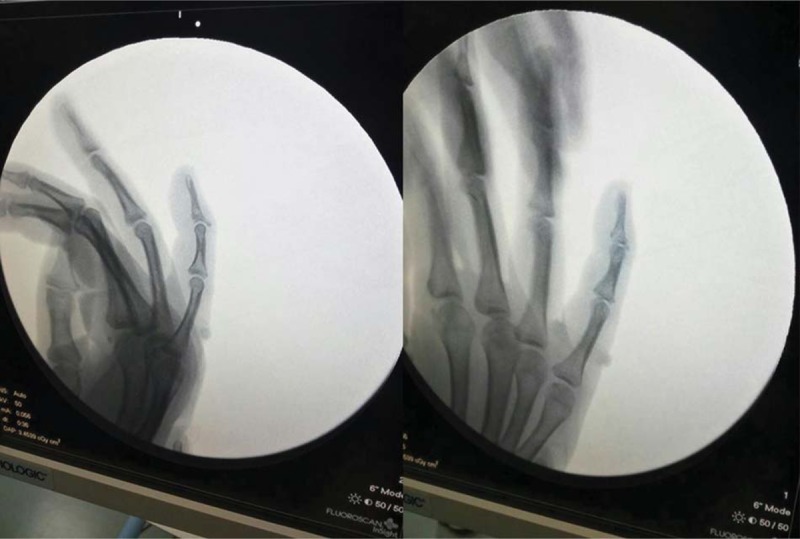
X-ray images were taken before the operation revealed that there is a avulsion fracture of more than one-fourth of the base located on the distal intra-articular.
